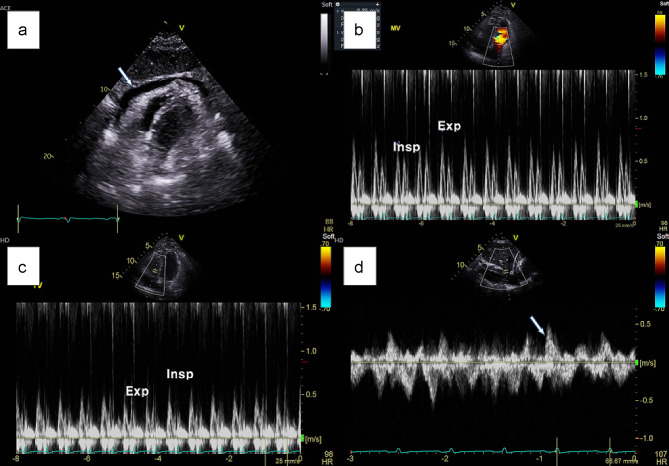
Transthoracic echocardiogram showing constrictive physiology. (a) Pericardial thickening and organized pericardial effusion are observed anterior to the right ventricular wall (arrow). (b) Mitral inflow velocity varies with respiration. (c) Tricuspid valve velocity varies with respiration. (d) Hepatic vein diastolic reversal flow with expiration is observed on pulse wave Doppler imaging (arrow)
